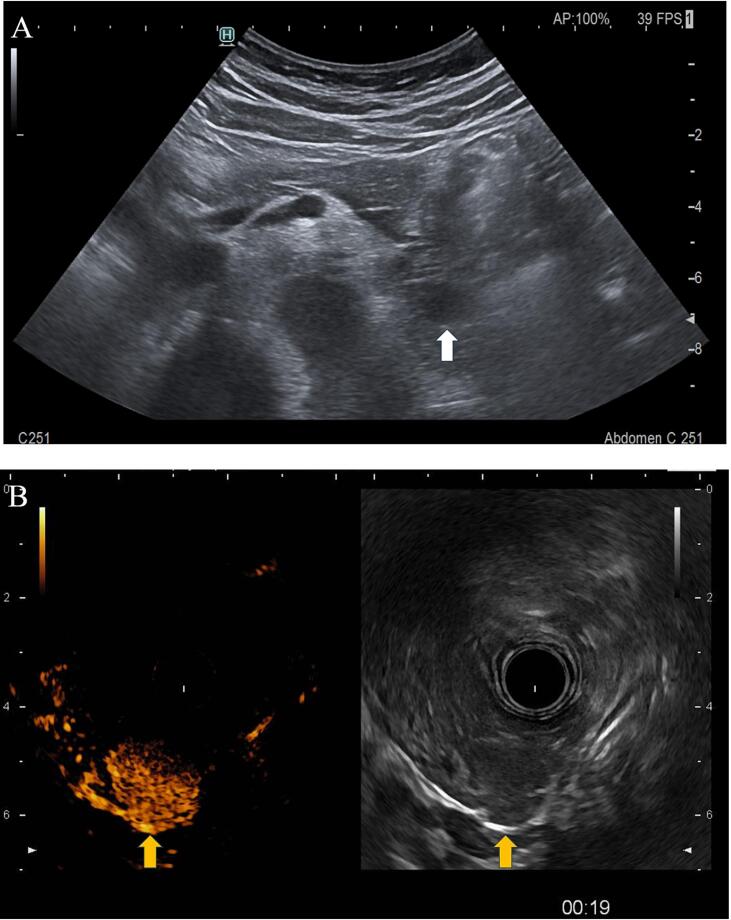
(A) Transabdominal ultrasound showing the insulinoma in the distal pancreas (white arrow). (B) Contrast-enhanced EUS showing insulinoma (yellow arrow). (For interpretation of the references to colour in this figure legend, the reader is referred to the web version of this article.)
